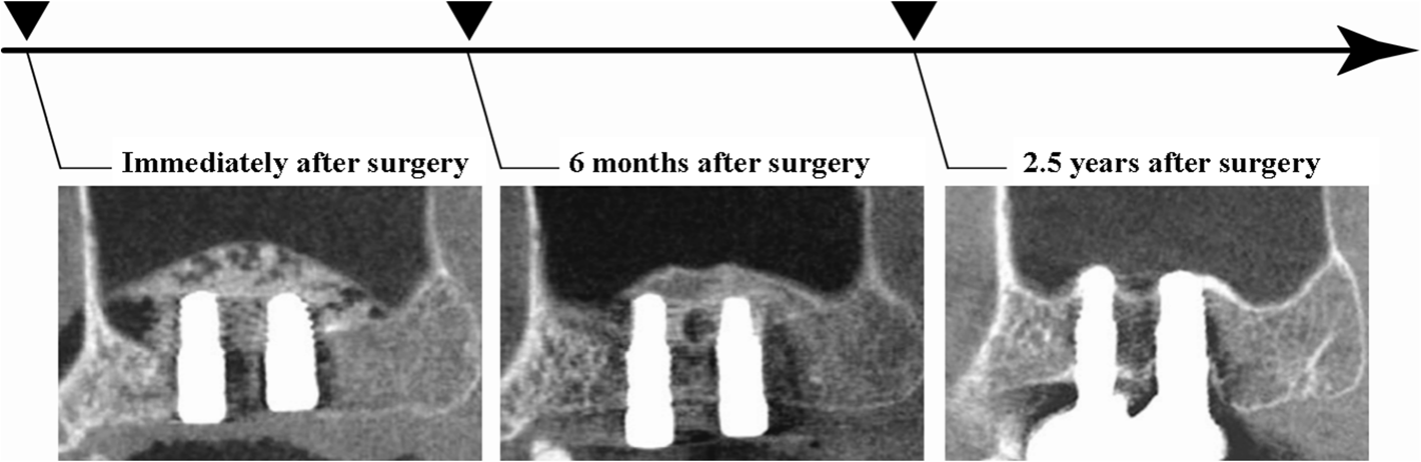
Treatment protocol for the present study. Postoperative CBCT was performed a minimum of three times, i.e., immediately, 6 months, and 2.5 years after implant placement
